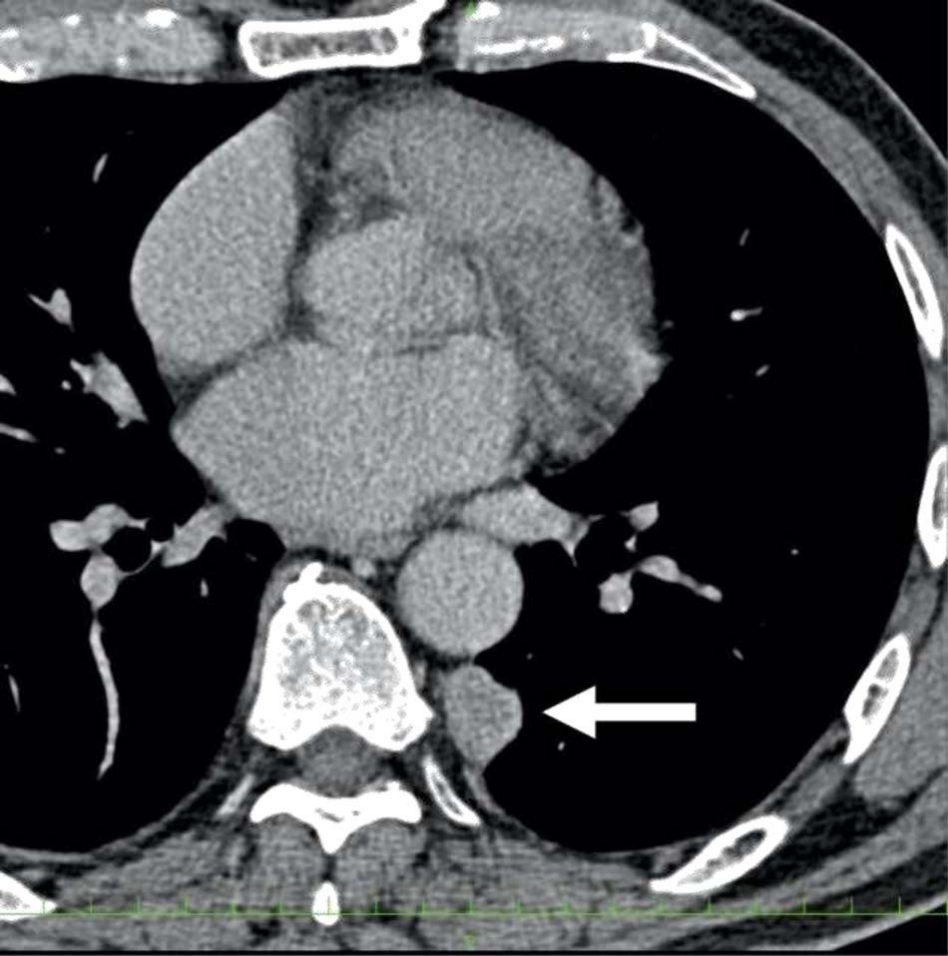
The computed tomographic findings of the primary tumor (arrow).
